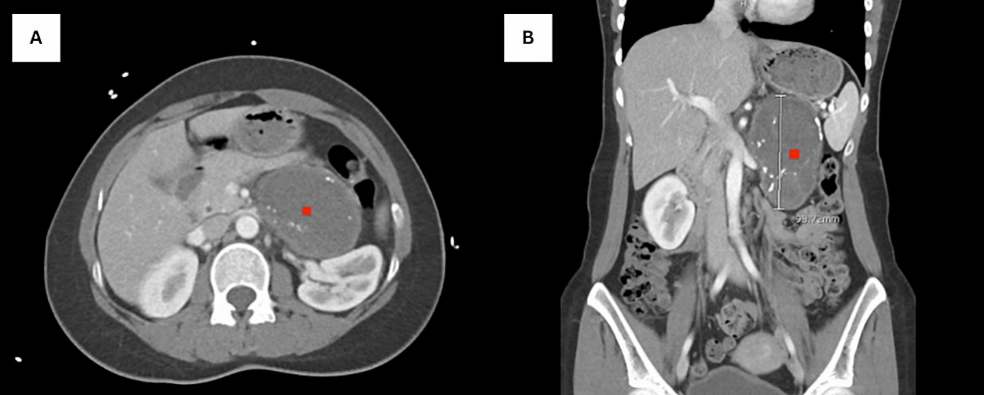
Computed tomography images (A) Clinical image showing axial computed tomography of well-defined, solid, encapsulated mass indicative of left adrenal ganglioneuroma (red square). (B) Coronal section of the left-sided adrenal ganglioneuroma around 98.72 mm in size (red square).
